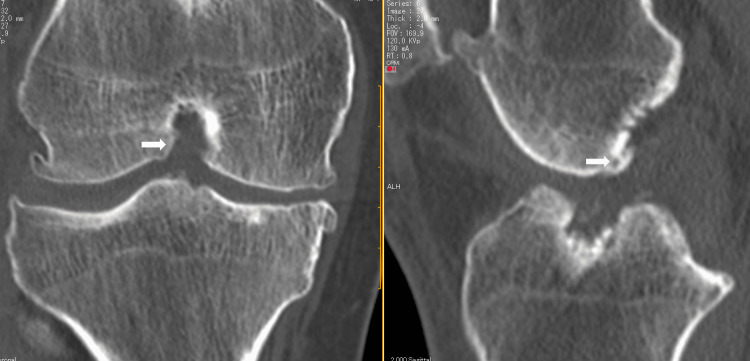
CT showed that the tibial bone hole is blocked by osteophytes outside the intercondylar notch (white arrow). CT: computed tomography
